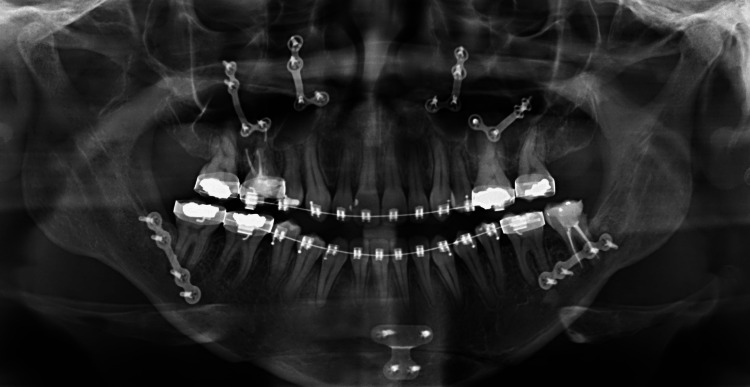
Post-operative panoramic radiograph. This is a panoramic radiograph of the patient after undergoing the orthognathic surgery which included Le Fort 1 osteotomy, bilateral sagittal split osteotomy, and advancement genioplasty.
